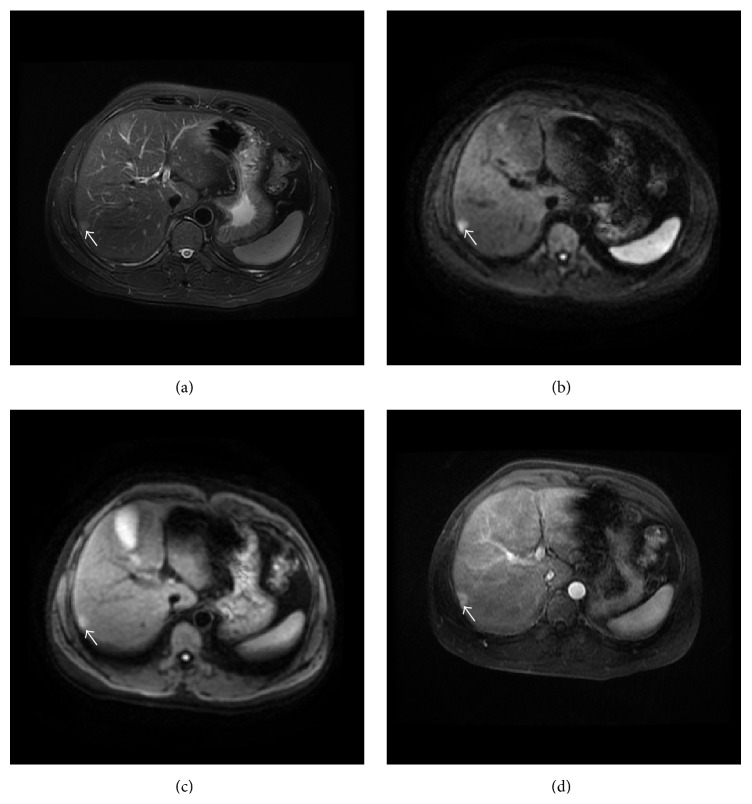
Transverse images in 49-year-old man with HCC. (a) Fat-suppressed T2-weighted fast spin-echo image shows that lesion is slightly hyperintense to the liver. (b) DW image with higher *b*-value shows that lesion is strongly hyperintense to the liver. (c) DW image with low *b*-value shows that lesion is slightly hyperintense to the liver with ill-defined margin. (d) Contrast-enhanced T1-weighted image in arterial phase demonstrates strong enhancement of lesion.
